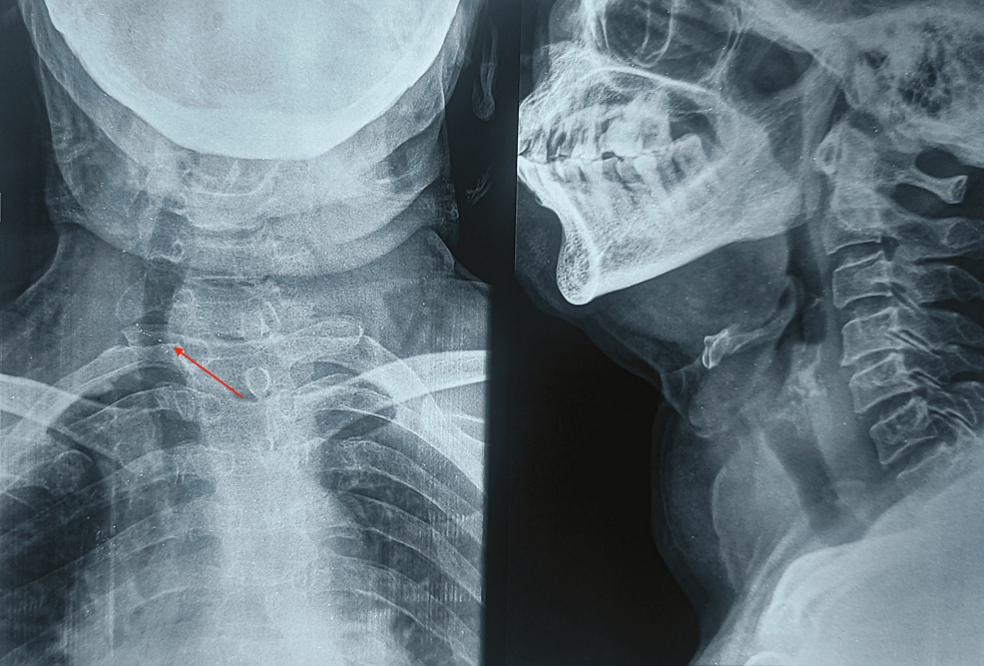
X-ray of the neck showing deviation of trachea towards right side
